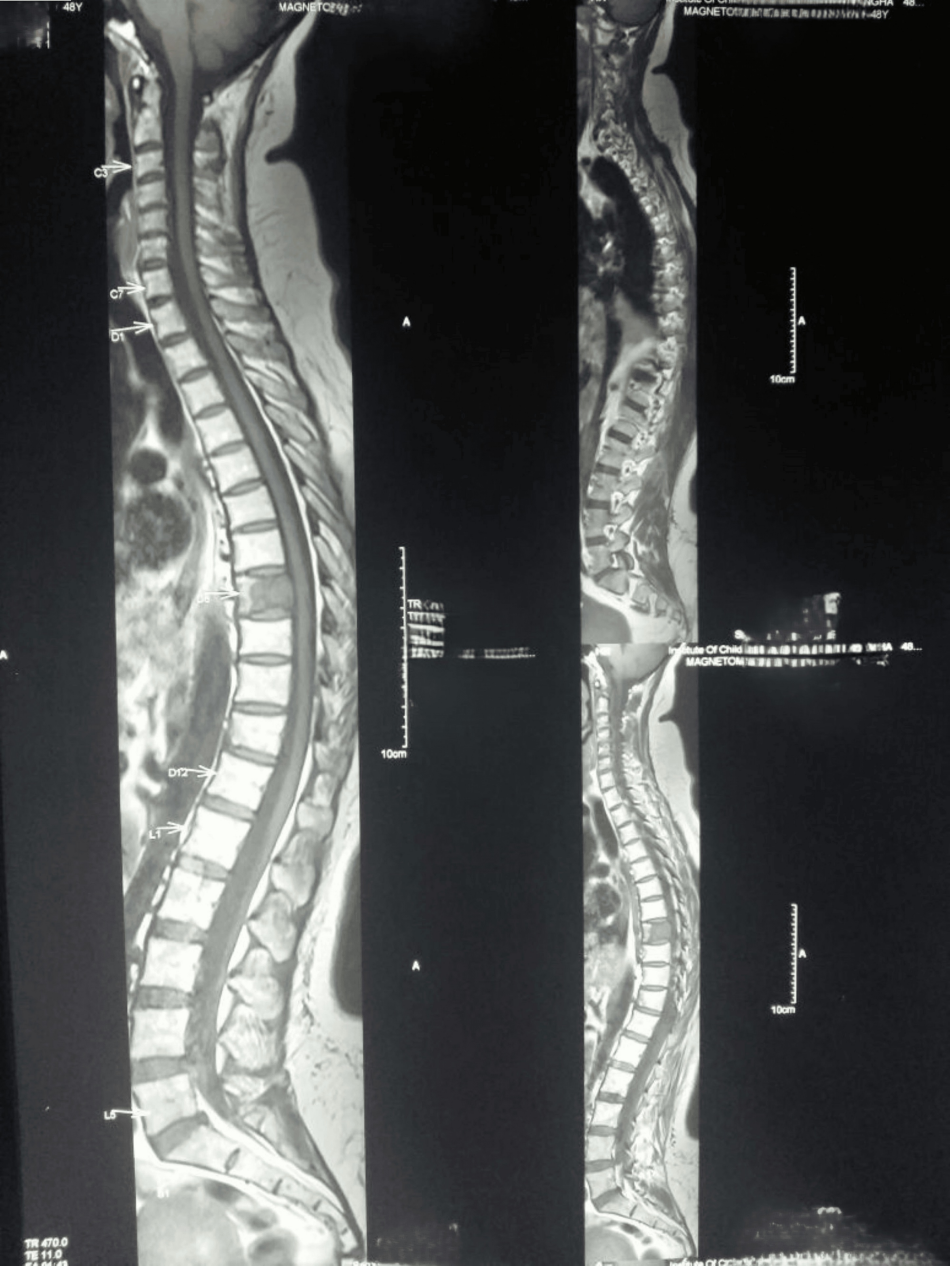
MRI showing solitary metastasis in D8 D8: eighth dorsal vertebra
